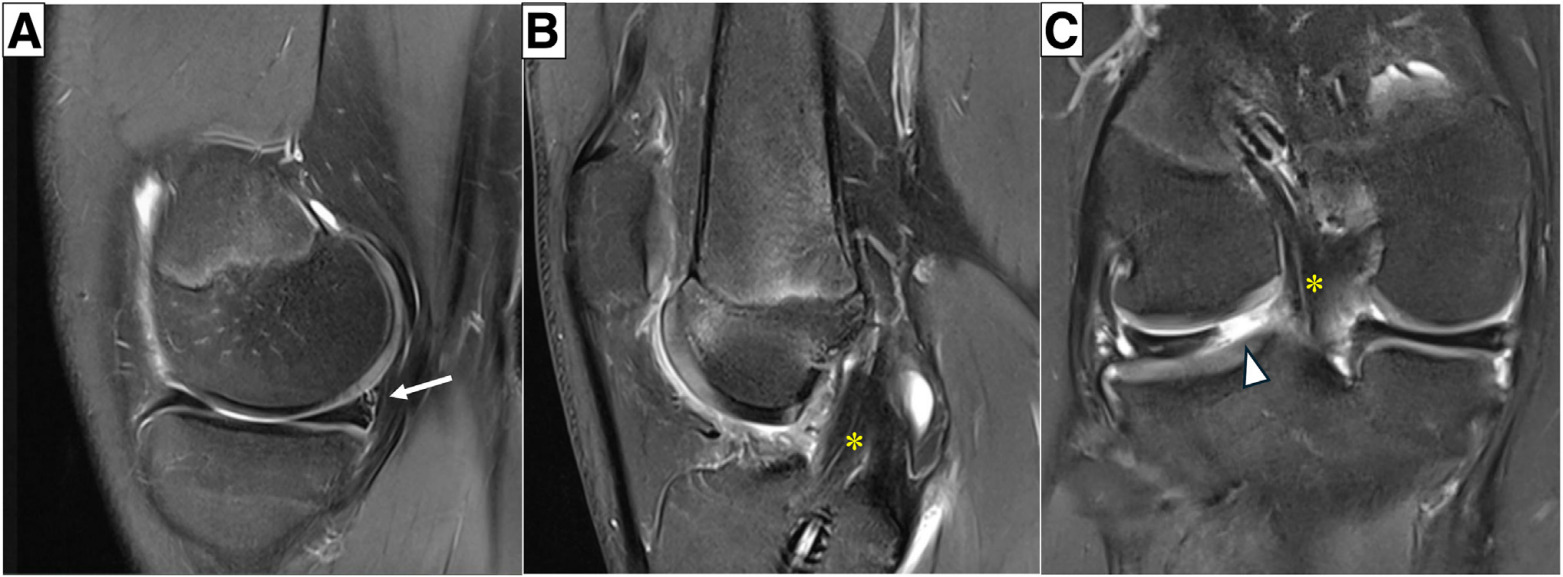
(A) Preoperative magnetic resonance image shows ramp lesion of medial meniscus (white arrow). (B) Previous reconstructed graft is still visible (∗), but tibial bone tunnel was misplaced posteriorly. (C) Coronal view shows posterior root radial tear of the lateral meniscus (arrowhead).
